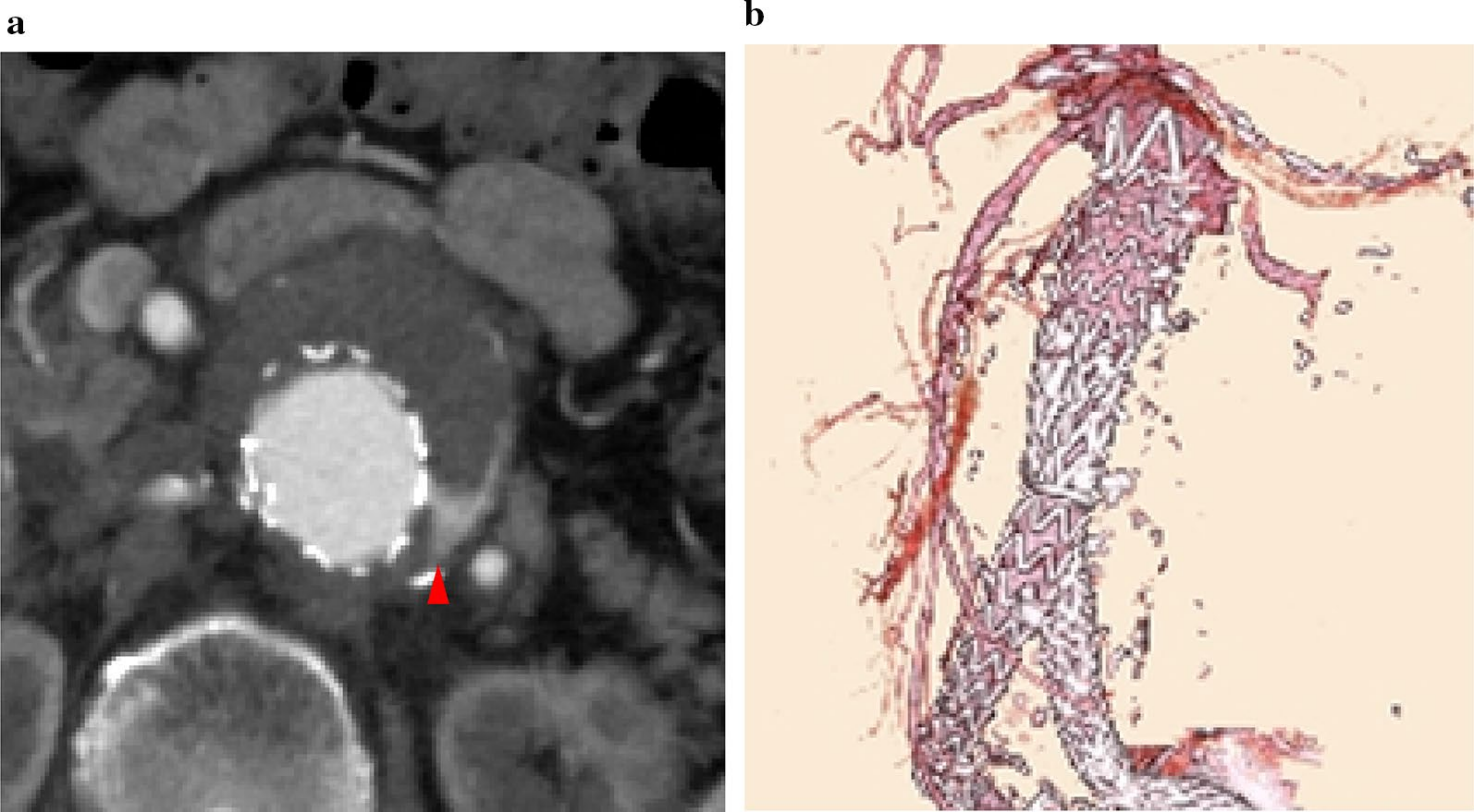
a The axial image of the postoperative computed tomography revealed a type Ia endoleak (arrowhead). b The 3D image of the postoperative computed tomography revealed a migration of the stentgraft
